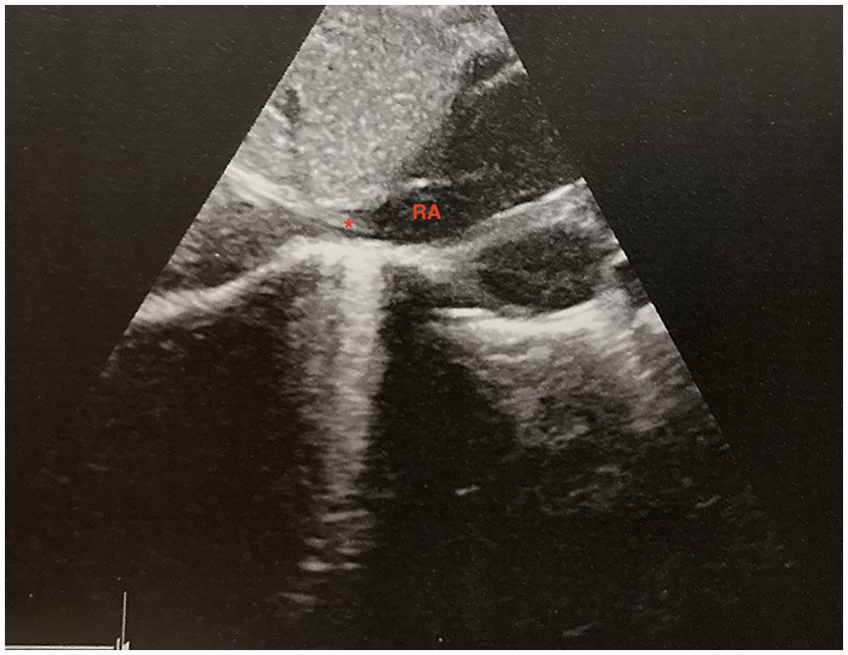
Ultrasound image of the correct placement of the umbilical venous catheter during a saline solution flush. RA, right atrium. * Catheter's tip.
